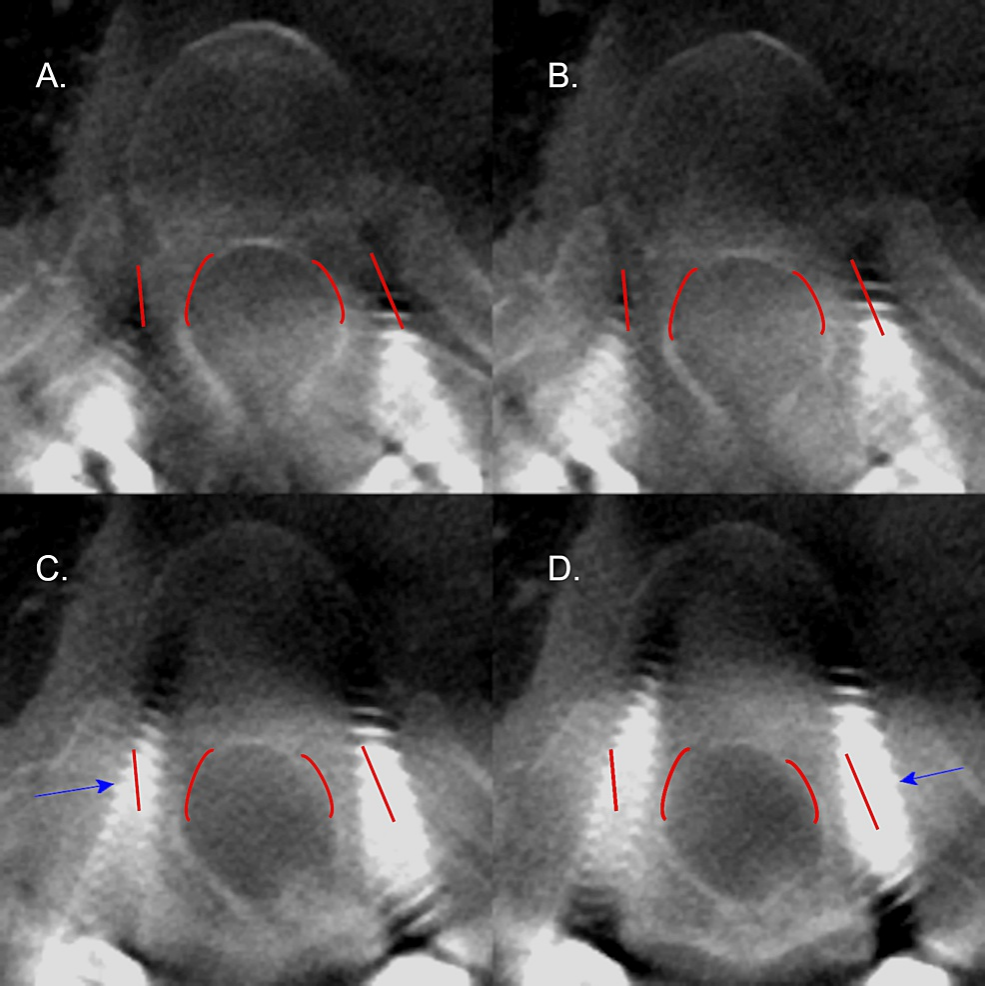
Axial CT radiographs of the T7 pedicle screws on patient 13. Axial CT radiographs demonstrating the T7 pedicle screws on patient 13 with an in-out-in (IOI) trajectory. Screw placement progresses from A to D. The pedicle of interest is outlined in red. The blue arrow denotes the largest deviation of the screw. Please note all CT images are in radiographic orientation.
